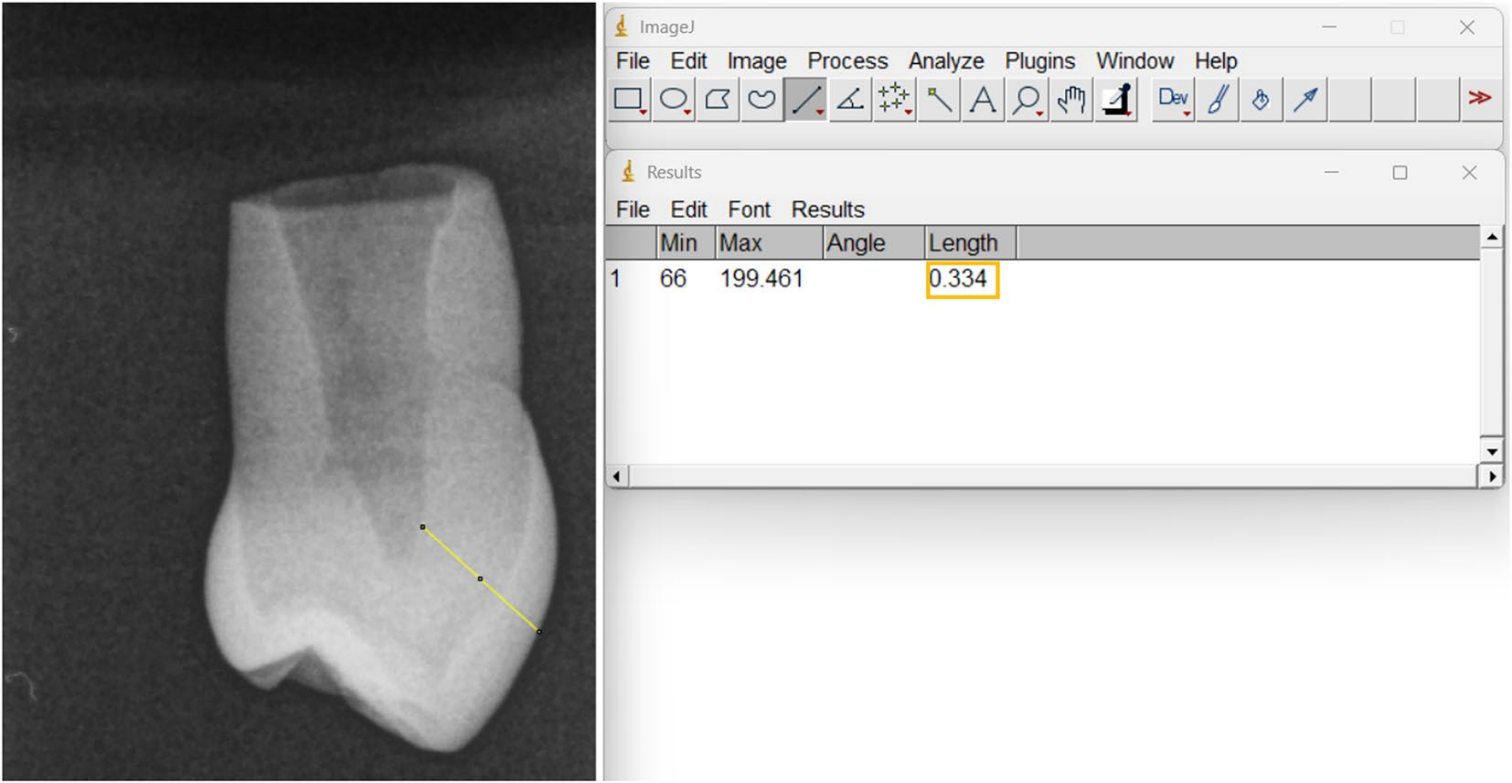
Measurement of buccal thickness in a premolar tooth. The left panel shows the digitized radiographic image, with the buccal thickness indicated along the white reference line. The right panel displays the corresponding measurement value, expressed in centimeters (cm).
